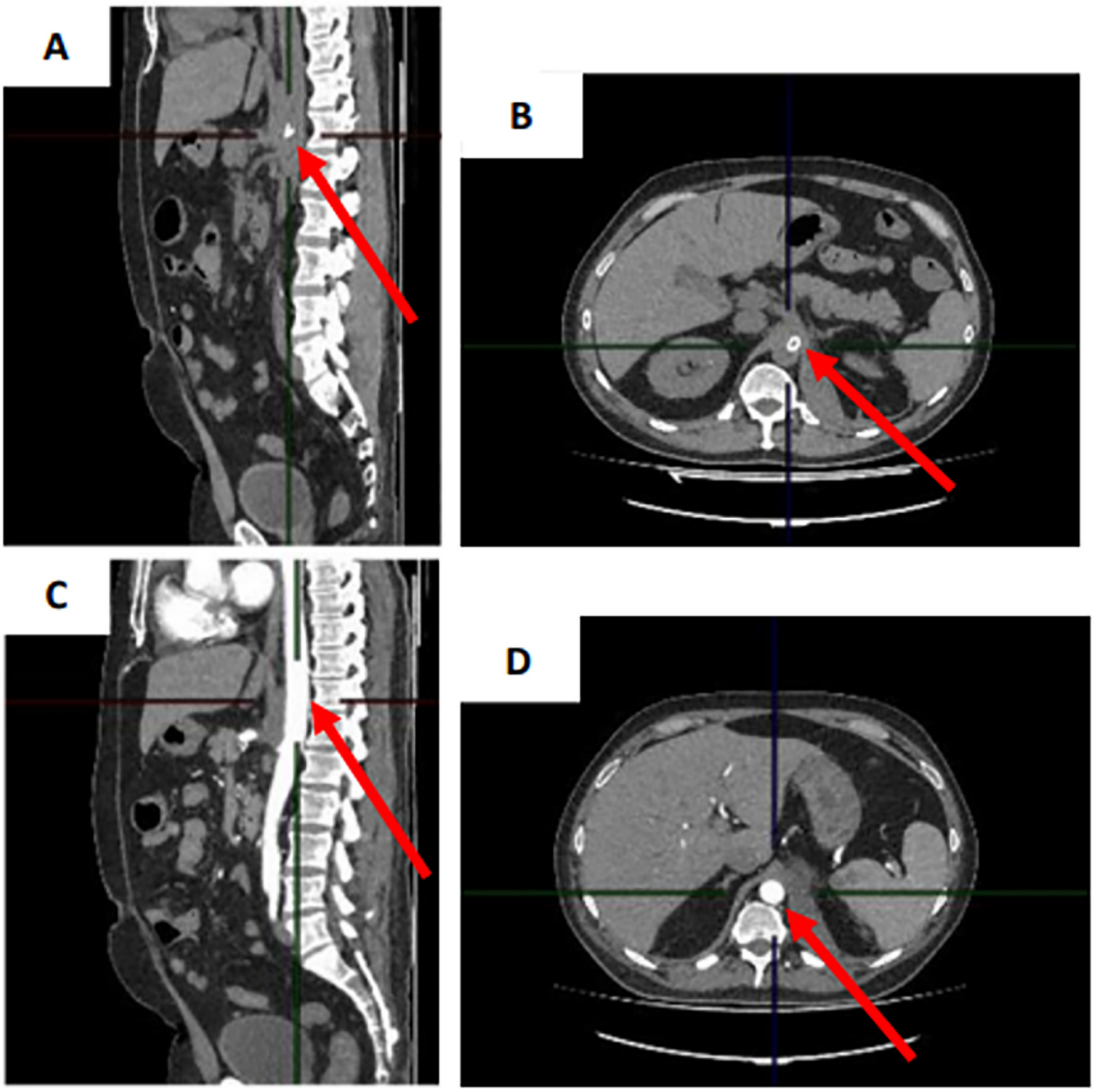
(A,B) CT scan of the abdomen with intravenous contrast in sagittal and transverse projection and (C,D) CT angiography scan of aorta and its branches with intravenous contrast in sagittal and transverse projection 3 weeks after stent graft implantation.
